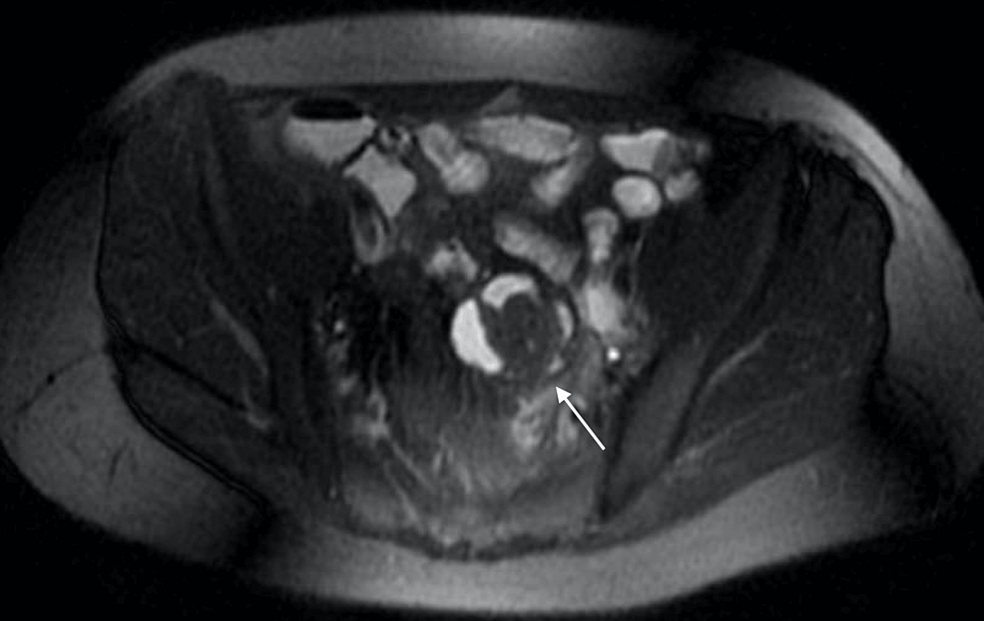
MR enterography with the axial T2-weighted image demonstrating infiltrating mass-like tissue (arrow).
